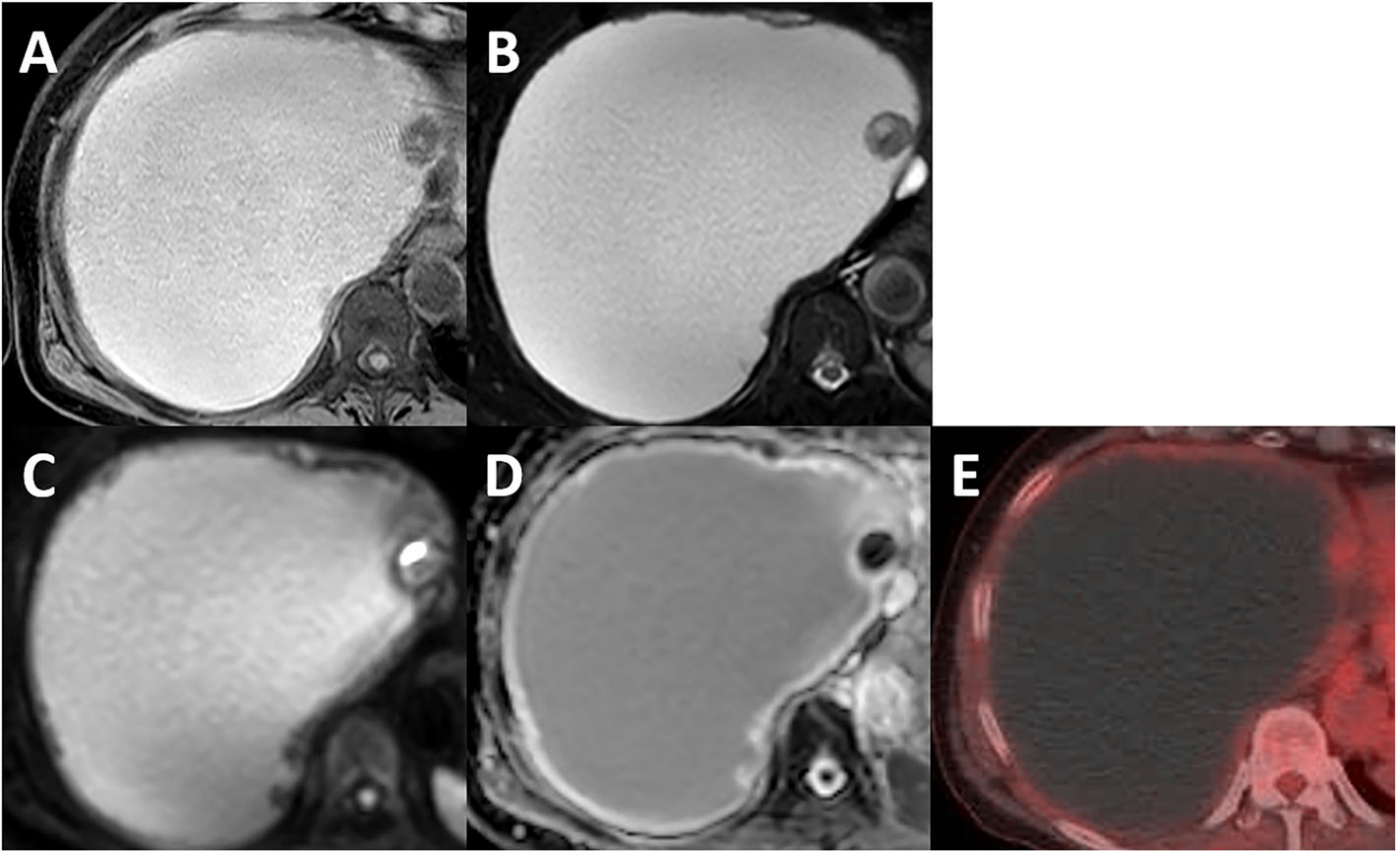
MRI and FDG-PET images of the ventral mural nodule. a MRI showed that the cyst content was a high-signal and the mural nodule was a low-signal on T1 W1. b MRI showed the cyst content was a high-signal and the mural nodule was partially high-signal on T2WI. c MRI showed that the mural nodule was partially strong high-signal on DWI, d with low ADC value (ADC_min_ 0.00 mm^2^/s, ADC_max_ 0.91 mm^2^/s, ADC_mean_ 0.6 × 10^−3^ mm^2^/s). e FDG-PET showed an abnormal uptake in the mural nodule with SUV-max 2.3
